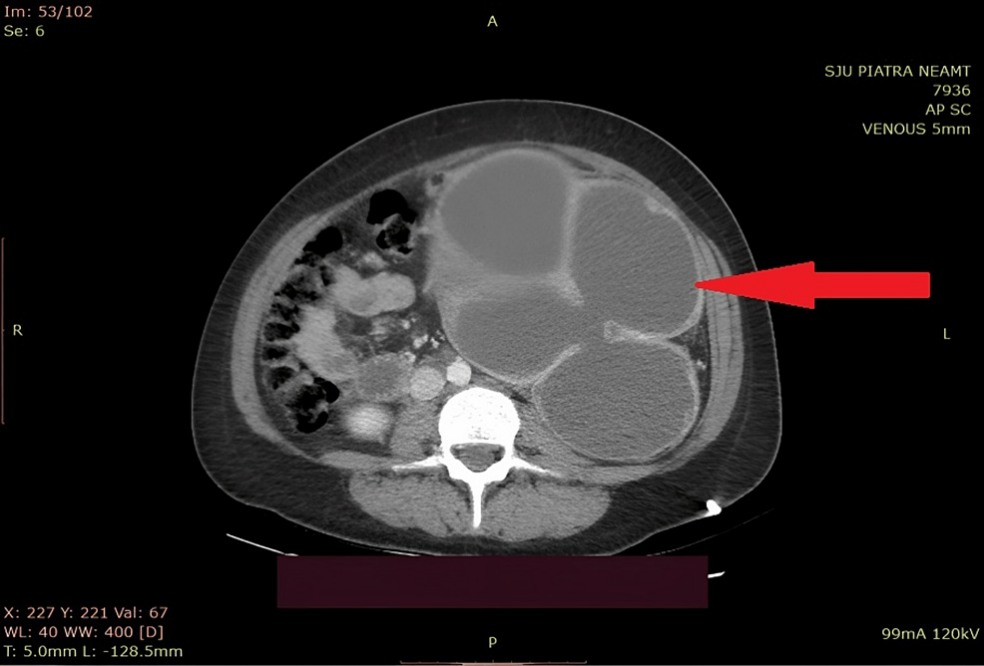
The CT scan showing the left kidney as a huge cystic mass
